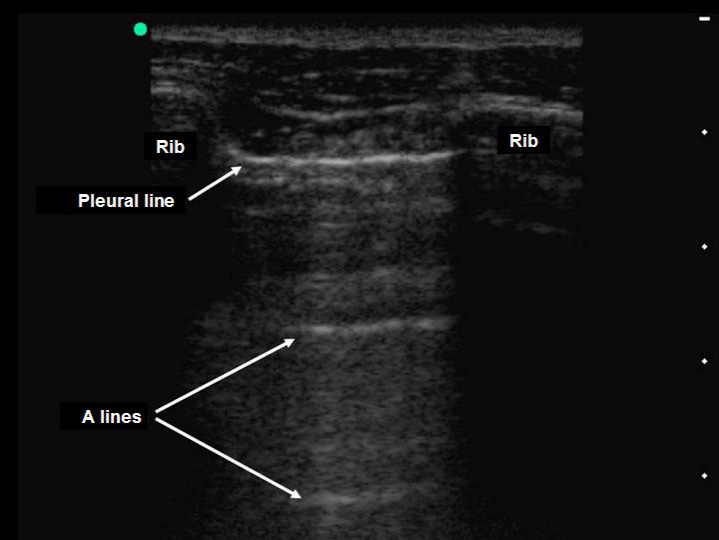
Pleural ultrasonography in two-dimensional mode [31]. The pleural line is seen between two ribs. Lung sliding is abolished when both the parietal and visceral pleura do not slide while the patient is breathing. The A-line sign corresponds to the presence of linear horizontal artefacts at regular intervals below the pleural line (A-lines) without B-lines. The A-line sign is part of the ultrasound semiology of the normal lung and pneumothorax. Reproduced with permission (ACCP - Chest).
